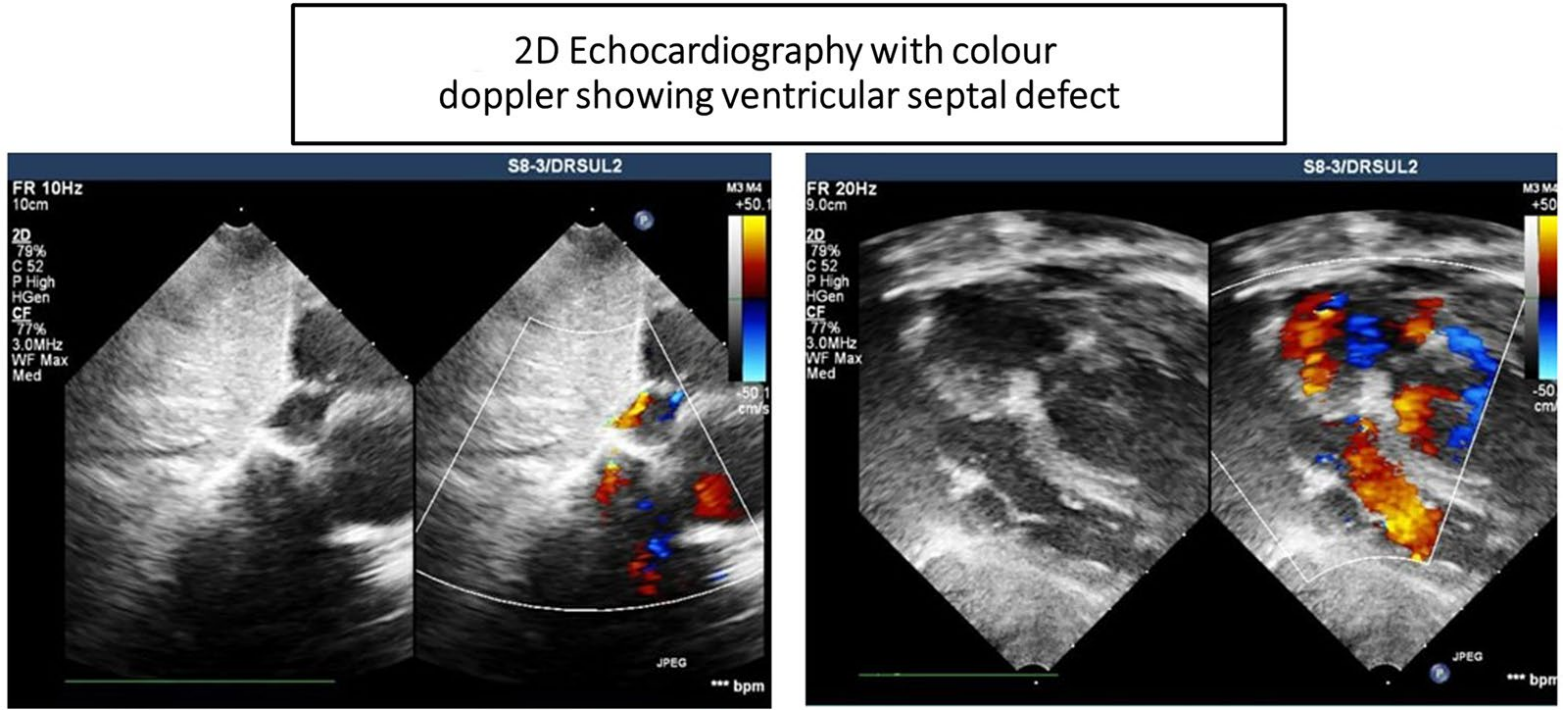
Two-dimensional echocardiography with color Doppler showing ventricular septal defect
